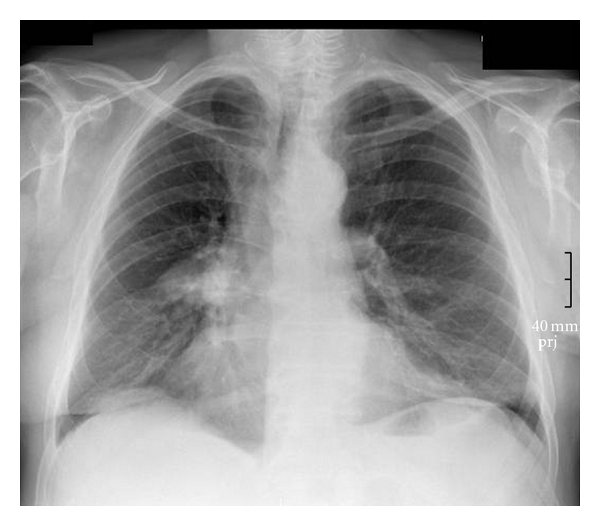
Chest radiograph one month after bronchoscopic resection.
